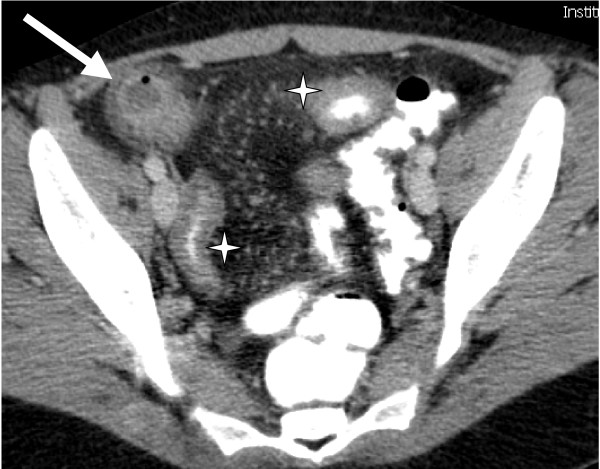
Preoperative Computed Tomography scanner showing a 3 × 3 cm abscess in right iliac fossa (arrow) as well as extensive inflammation of the terminal ileum (*).
